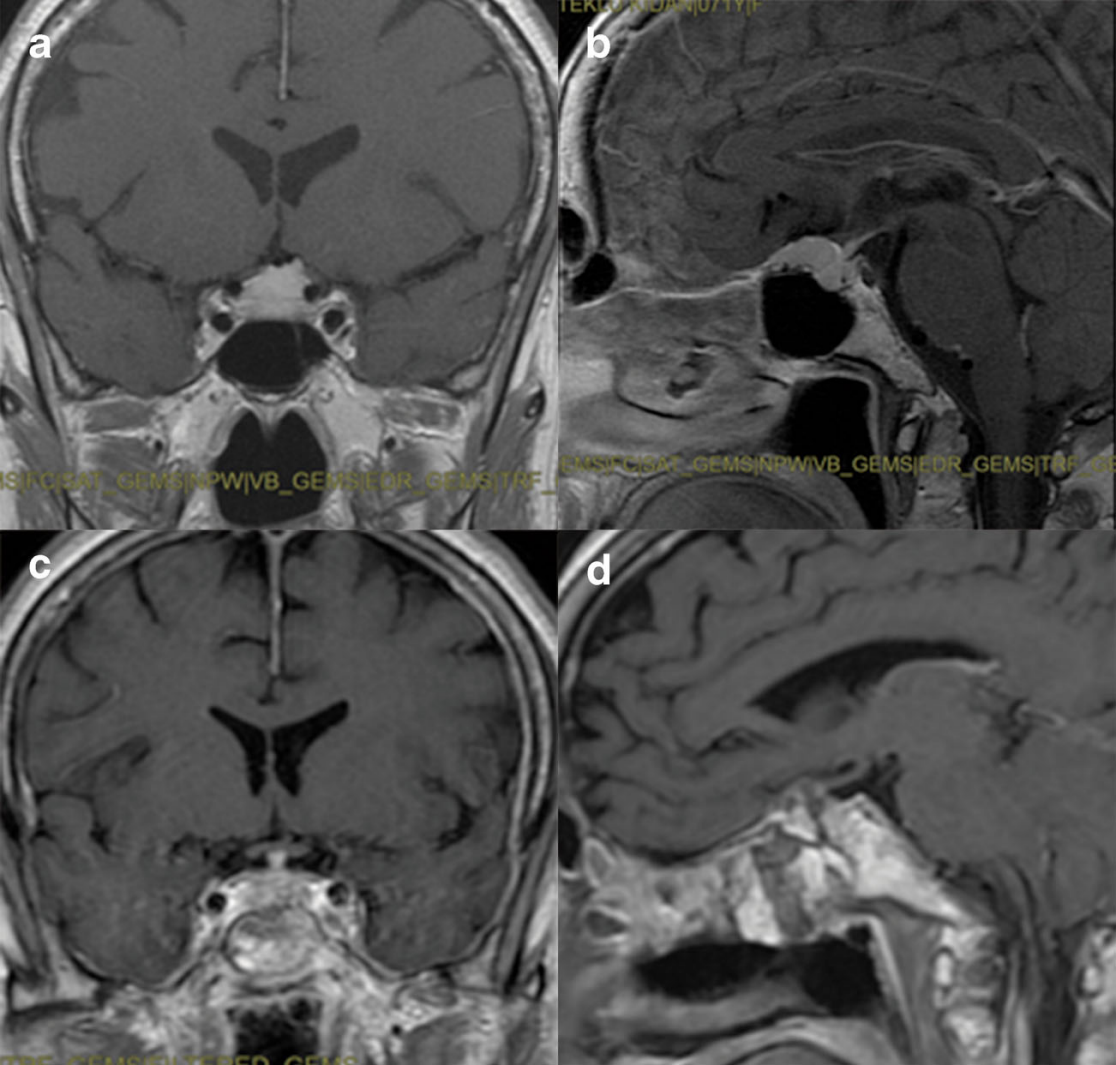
Post-contrast T1-weighted brain MRI, coronal and sagittal view, showing preoperative (a, b) and postoperative (c, d) images of a tuberculum sellae meningioma
